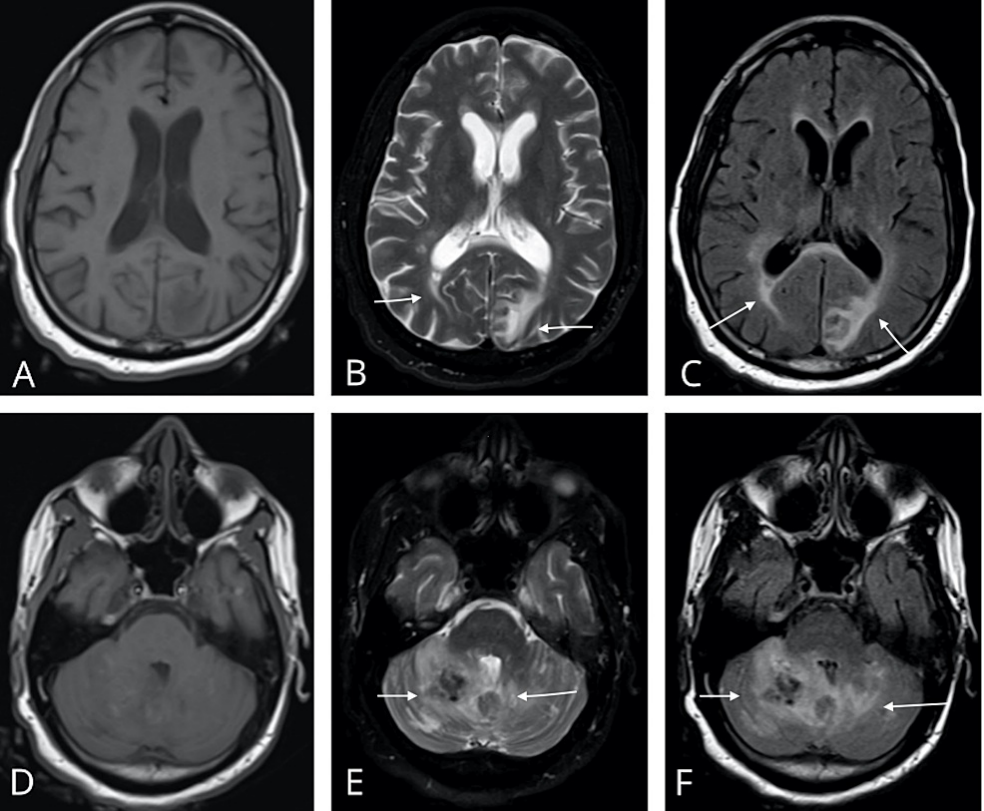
Magnetic resonance imaging of the brain in axial view demonstrating PRES in the patient. (A) T1-weighted MRI reveals no abnormalities in the cortex. (B) T2-weighted MRI reveals bilateral hyperintense regions of the periventricular and subcortical white matter of occipital lobes. (C) FLAIR sequence reveals bilateral hyperintense regions of the periventricular and subcortical white matter of the occipital lobes. (D) T1-weighted MRI reveals no abnormalities in the cerebellar region. (E) T2-weighted MRI reveals an abnormal parenchymal mass in the vermis and right cerebellar lobe. (F) FLAIR sequence reveals an abnormal parenchymal mass in the vermis with hyperintense extension into the right and left cerebellar lobe.
